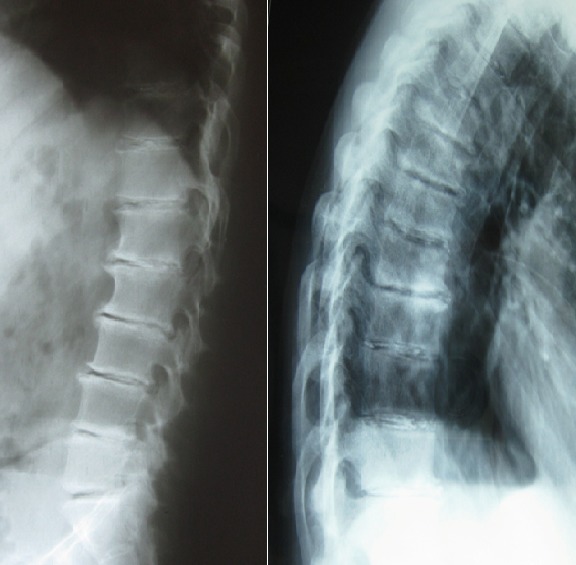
The standard radiographs of the lumbar spine (A) and dorsal spine (B) revealed multiple disc calcifications associated with large staggered disc clumps and discal empty images
